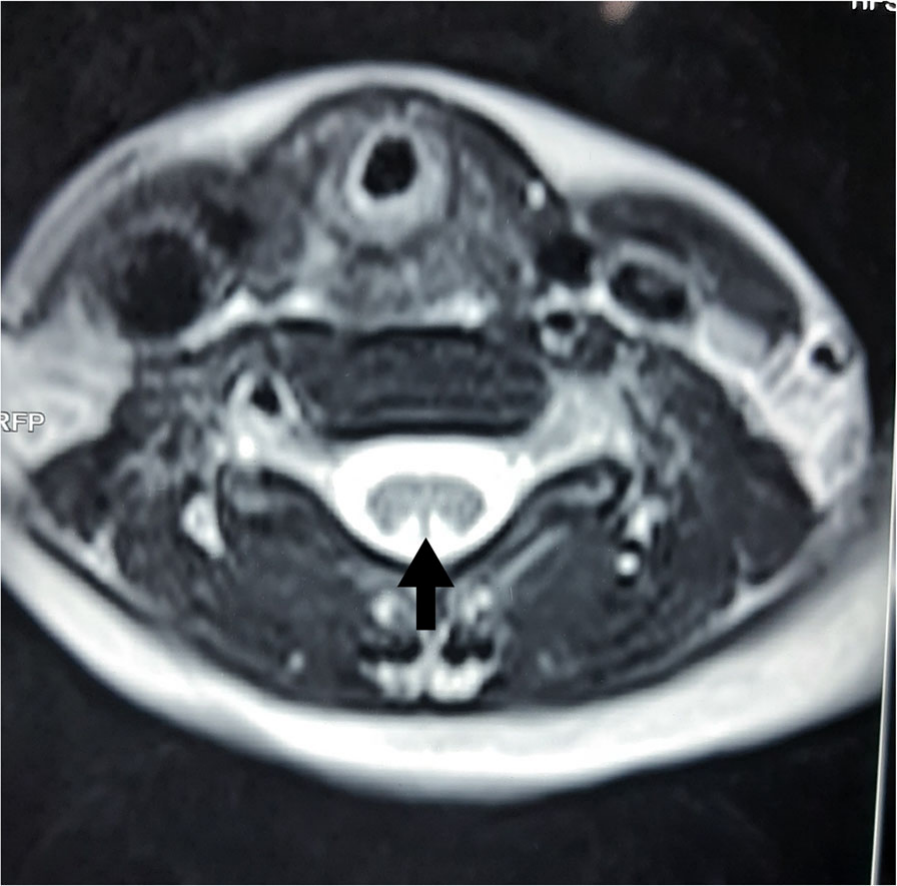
Axial T2-weighted MRI image showing symmetrical bilateral hyperintense signal in posterior columns; the characteristic ‘inverted V sign.’
